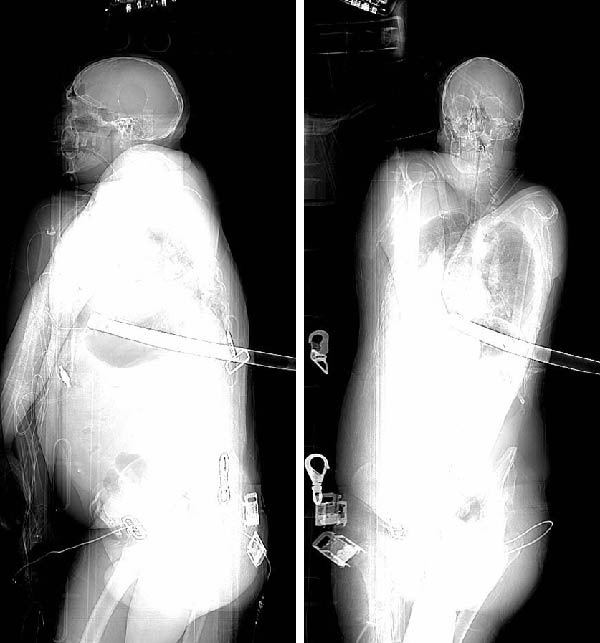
A computed tomography (CT) localizer radiograph was obtained before a full CT scan, and shows the potential scale of the injuries. An impaled object should be removed after complete CT scan with contrast, and under controlled conditions. Note that emergency thoracotomy without CT is indicated in selected unstable patients.
